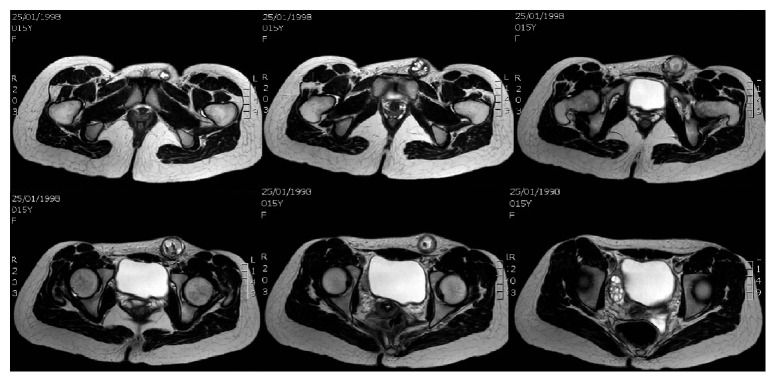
MRI study revealing the ovarian mass herniated in the inguinal region, transverse section.
